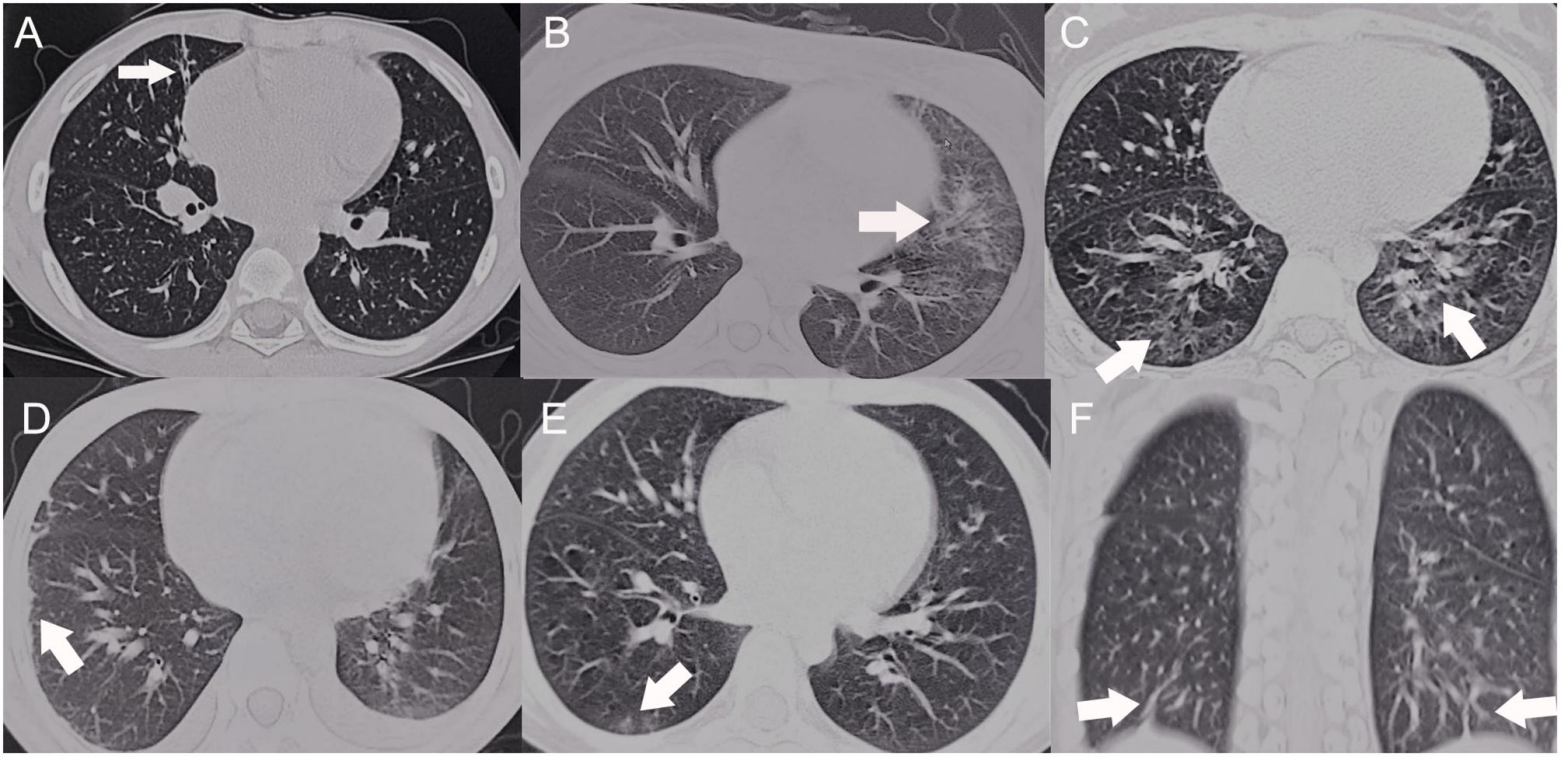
HRCT scans of the SLE patients with pulmonary involvement. (A) Fibrotic streak; (B) Reticular pattern; (C) Mosaic perfusion; (D) Pleural thickening (E) Ground glass opacity; (F) Subpleural interlobular septal thickening.
